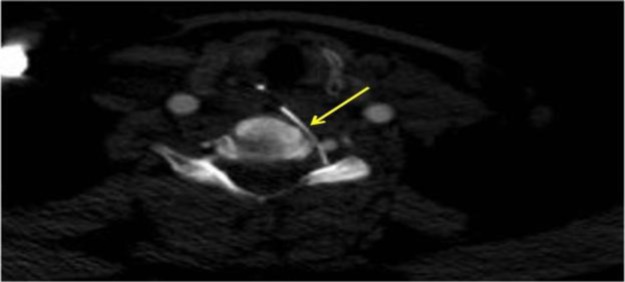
CT angiogram of the cervical spine showing transluminal migration of the foreign body through the esophagus, and its close relation to the left vertebral artery. The foreign body is depicted with a yellow arrow
